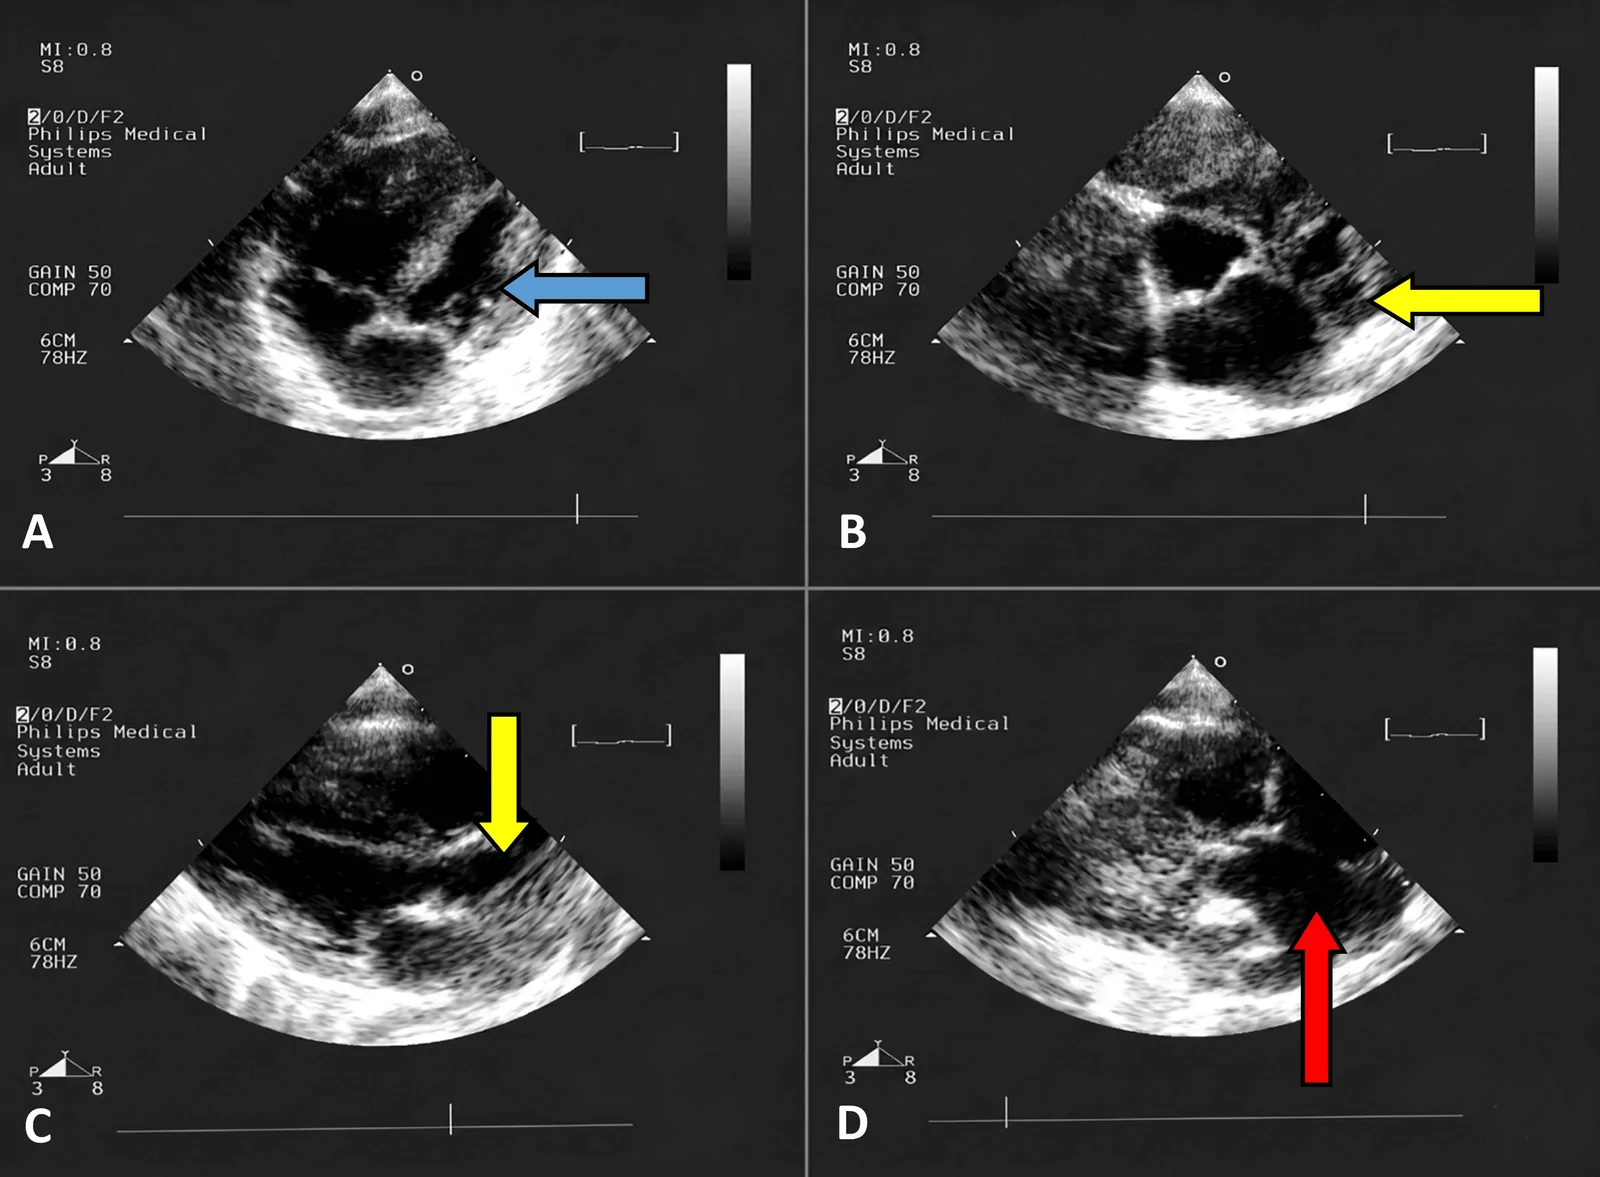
Preoperative transthoracic echocardiography of d-TGA anatomy. A: Apical four-chamber view demonstrating atrioventricular concordance alongside marked dilatation of the right-sided chambers as indicated by the blue arrow. B and C: Views depicting the classic parallel (non-crossing) outflow tracts, pathognomonic for dextro-transposition of the great arteries (d-TGA) as indicated by the yellow arrows. D: Parasternal short-axis view demonstrating a well-conditioned, circular ("O-shaped") left ventricle with no interventricular septal flattening indicated by the red arrow, confirming preserved ventricular geometry suitable for immediate systemic afterload.
